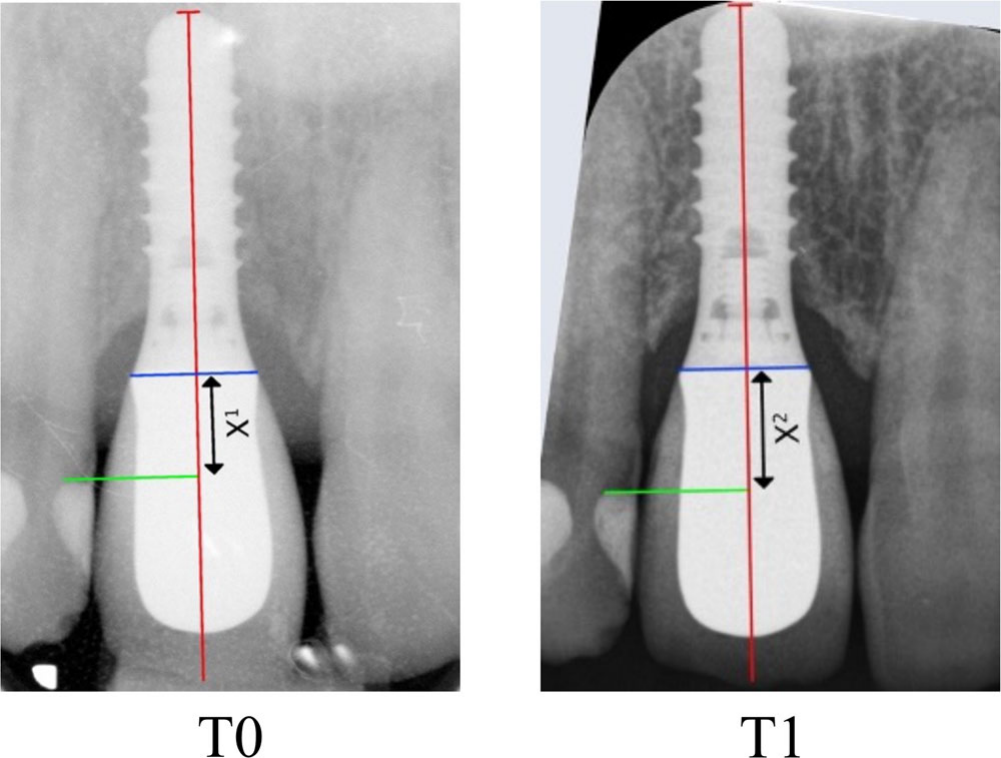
Radiograph taken at prosthesis placement (reference T0) and at the long‐term control (T1). The implant length was measured on each radiograph and compared to that given by the manufacturer for internal calibration. Reproducible landmark (green) on the adjacent teeth was selected on T0 and T1 radiographs. The projection of the T0 landmark (green) on the long axis of the implant only (red) and not the crown established the baseline distance X1. The same measurement was made on the T1 image. X2 was then subtracted to X1 to determine the amount of vertical discrepancy between T0 and T1.
